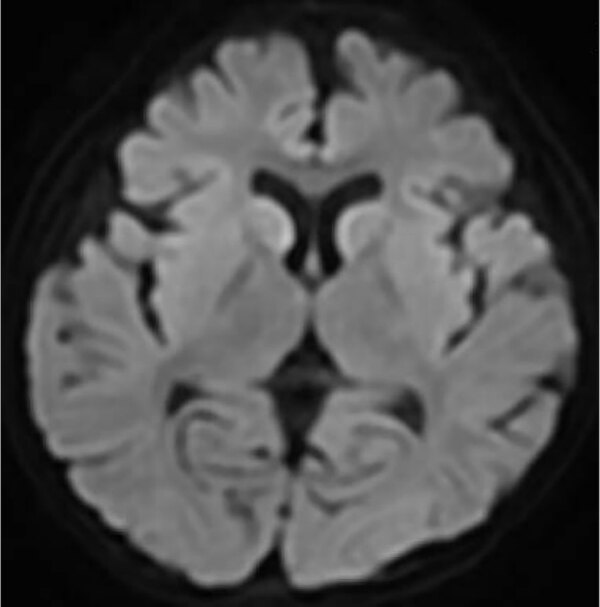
Diffusion weighted magnetic resonance imaging scan of brain in study of acute encephalopathy associated with human adenovirus type 14 infection in 7-year-old girl, Japan. Image shows high intensity in the subcortical white matter and bilateral caudate nuclei.
